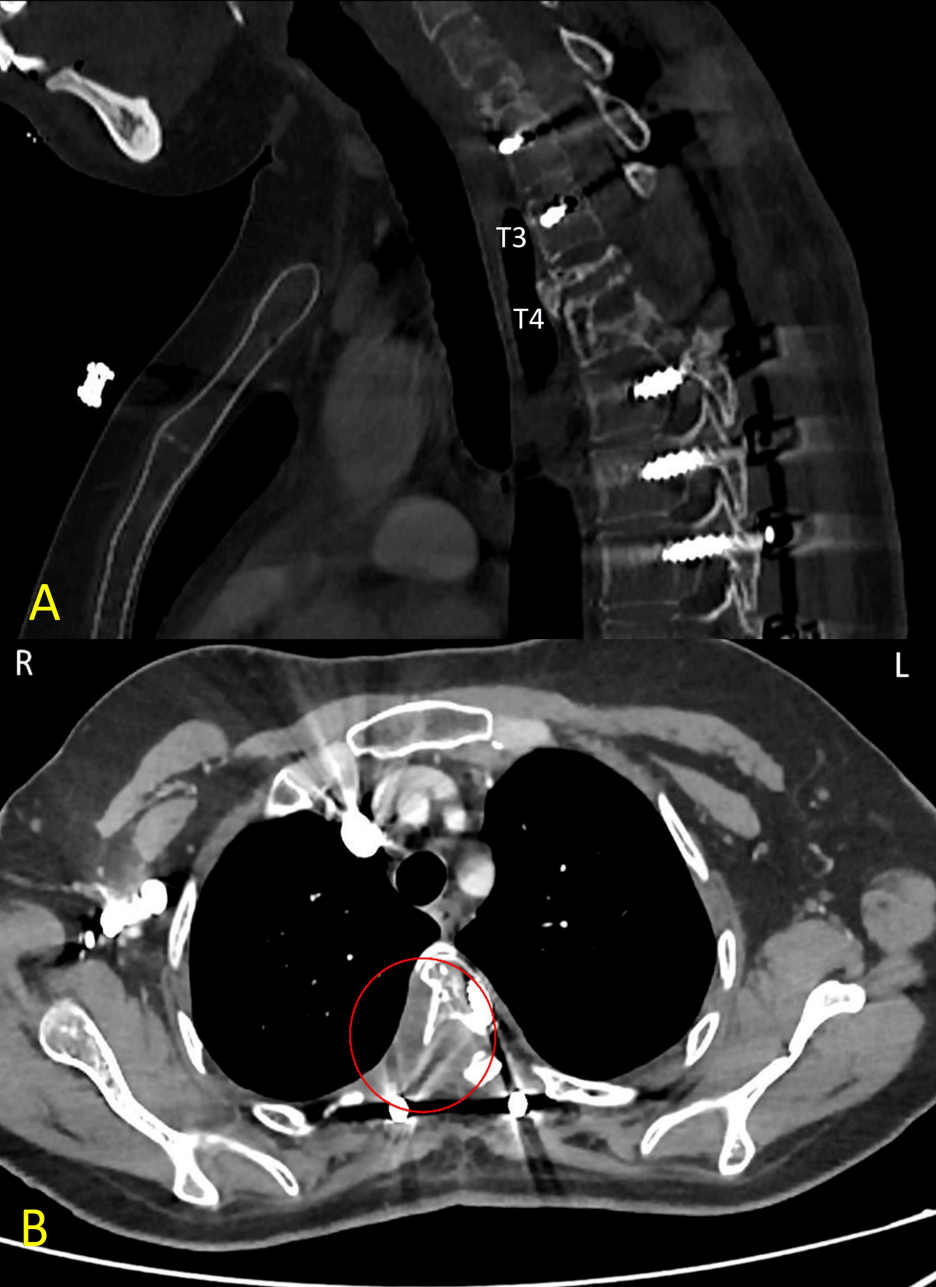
Sagittal and Axial Chest CT Scans (A) Sagittal chest CT showing destructive bone lesions in the T3 and T4 vertebral bodies with associated soft tissue component. (B) Axial chest CT demonstrating possible right-sided pleural invasion (circle) and involvement of adjacent structures. CT, computed tomography
